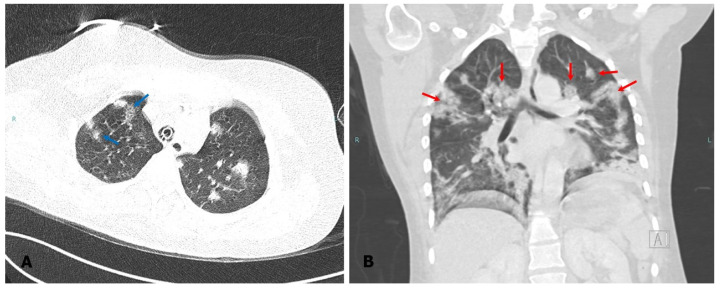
Computed tomography of the chest. The image taken prior to the diagnosis of fungal infection shows consolidation of ground glass opacities ((A), blue arrow) and bilateral nodular opacities in the upper lobes ((B), red arrow).
